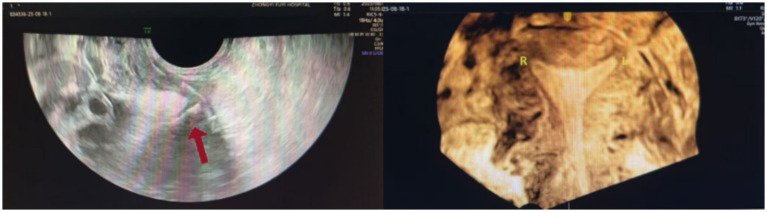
Preoperative ultrasound image.
